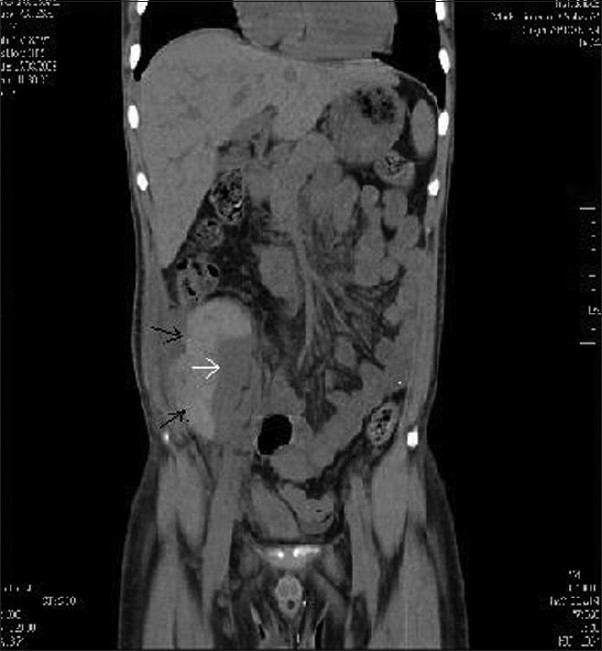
CT of the abdomen-(coronal section) showing sub capsular hematoma (black arrows) compressing the graft kidney (white arrow)
